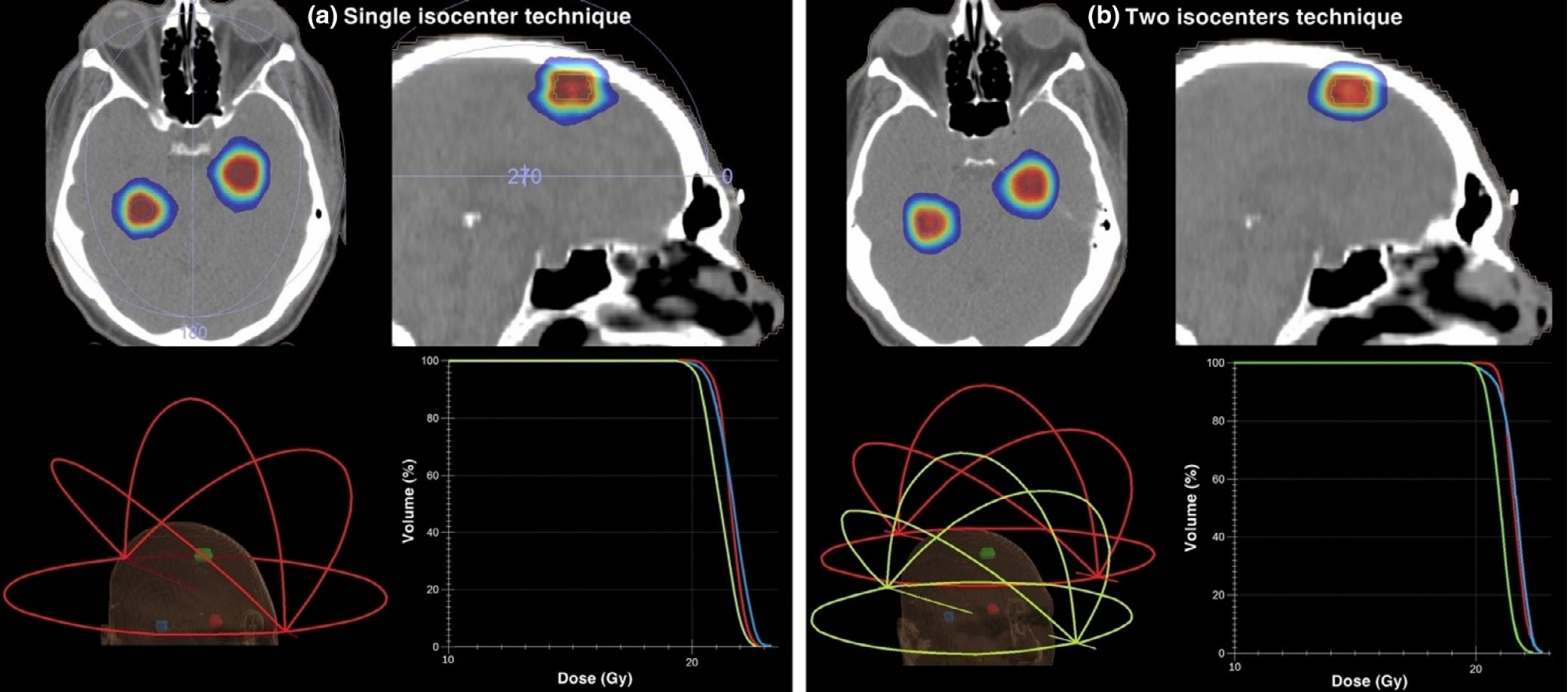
Indicative (patient #4) reference treatment plans prepared using both the (a) single‐ and (b) two‐isocenter techniques. Isodose lines, corresponding to reference dose distributions, are superimposed on axial and sagittal slices (top) of the planning CT scan. Arcs configuration and related DVHs are also presented (bottom).
